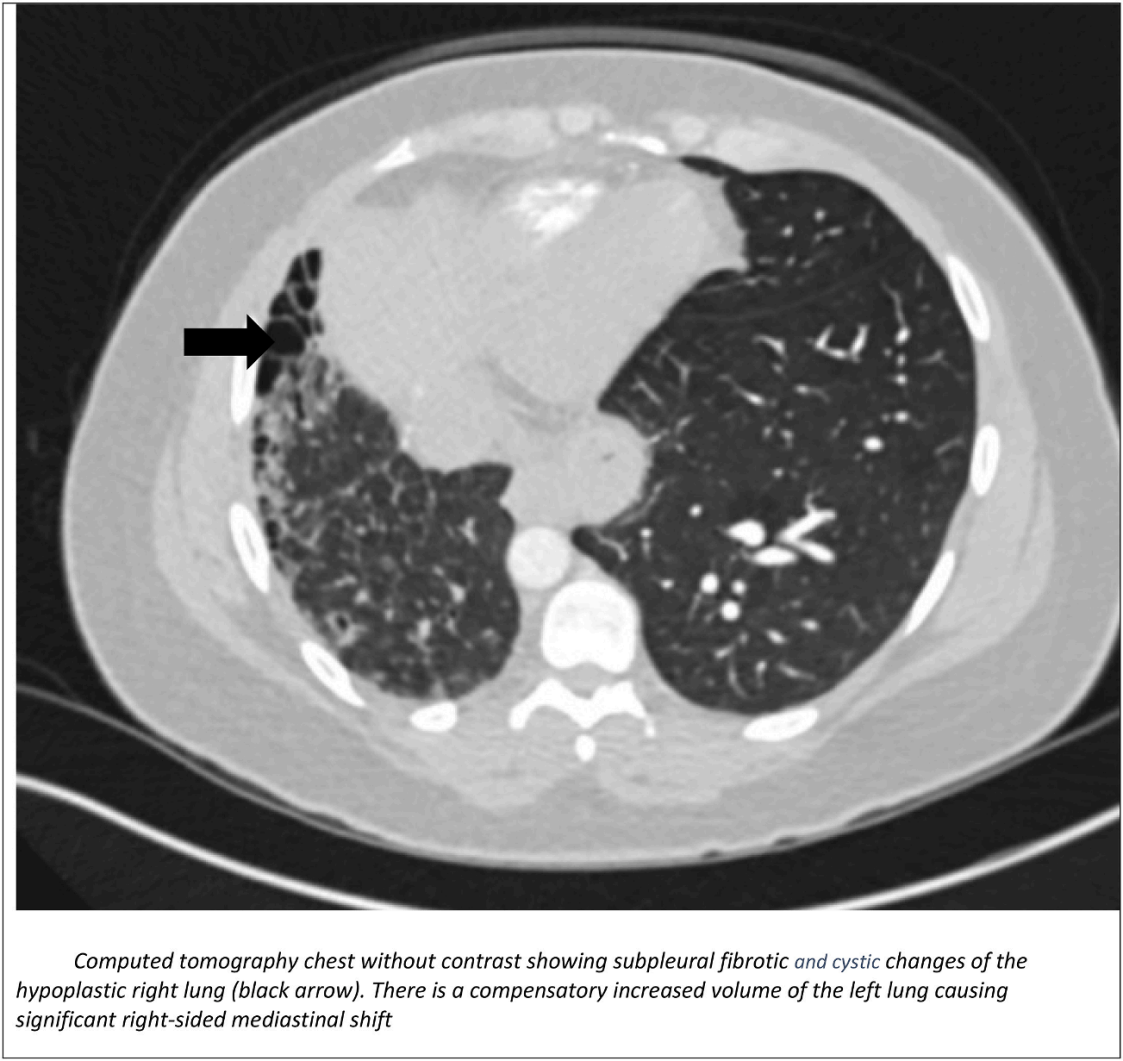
Computed tomography chest without contrast showing subpleural fibrotic and cystic changes of the hypoplastic right lung (black arrow). There is a compensatory increased volume of the left lung causing significant right-sided mediastinal shift.
